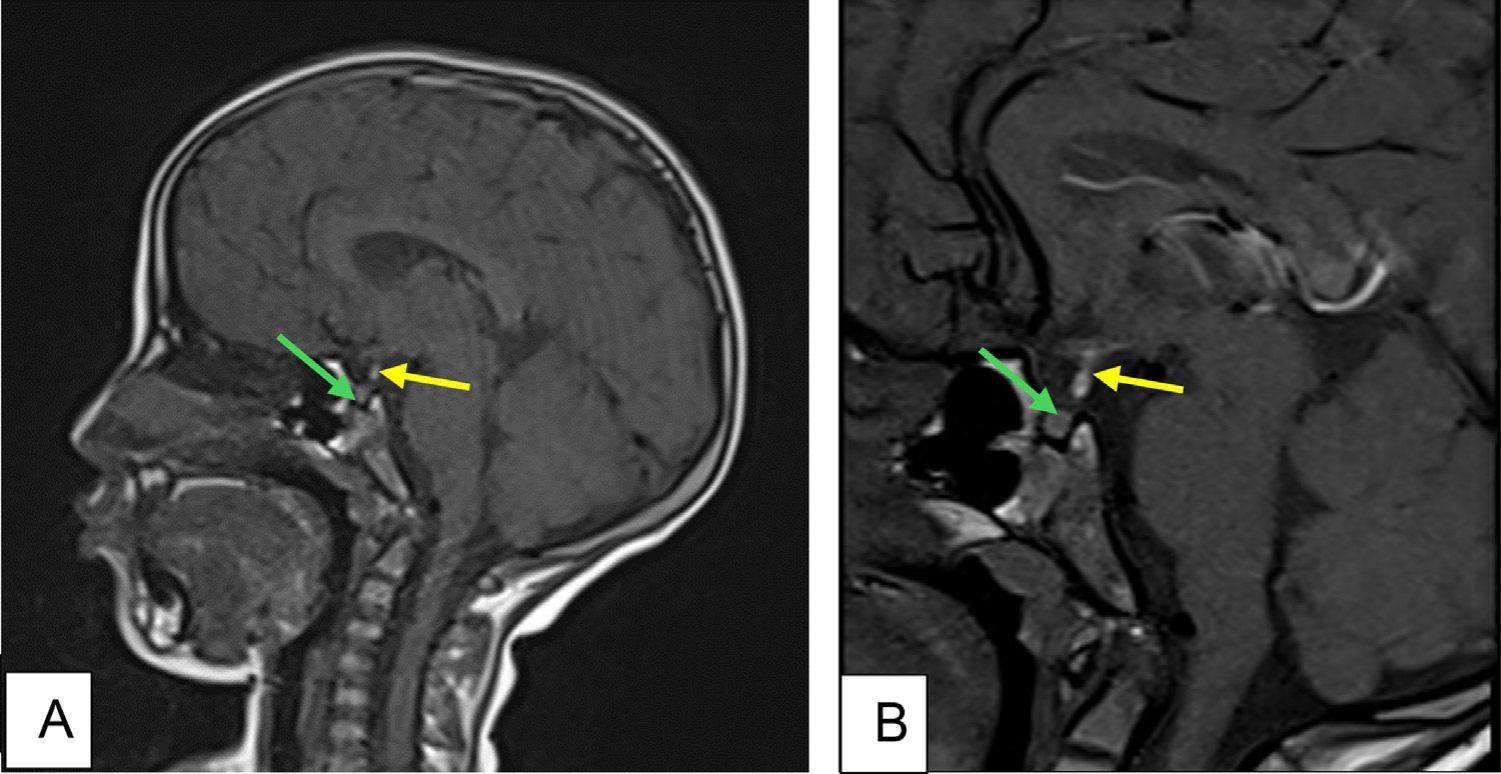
Pituitary MRI finding. (A) And (B) Sagittal Pre contrast and Post contrast T1 weighted images show a hypoplastic anterior pituitary gland (green arrow) measuring 2.5 mm (Normal: 4.5±0.6mm) with an ectopic posterior pituitary located at the distal end of the pituitary stalk (yellow arrow). Note the absence of the identifiable pituitary stalk.
